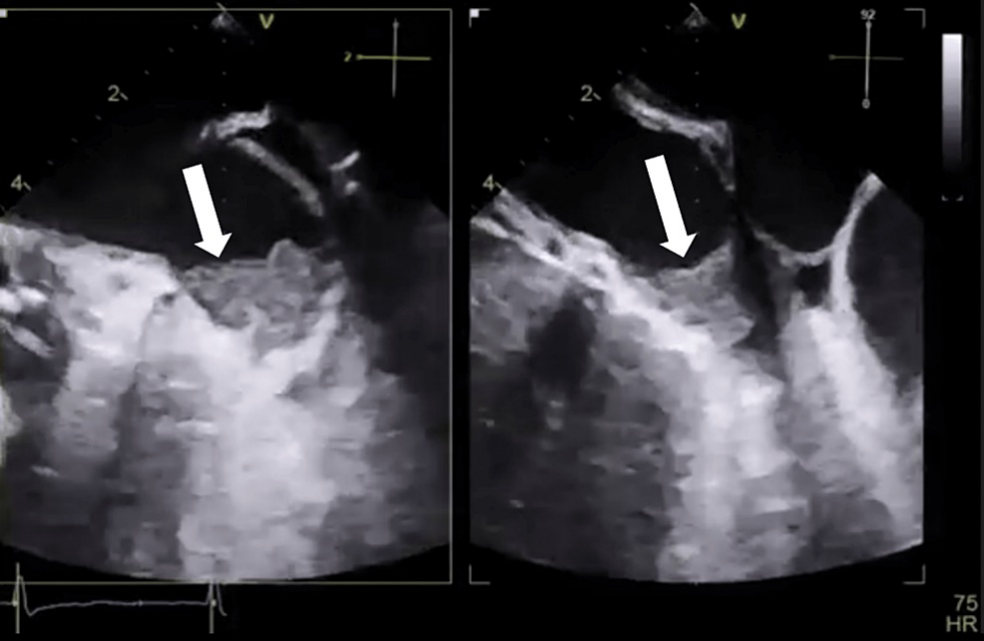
Transesophageal echocardiography mid-esophageal left atrial appendage view. Note the large thrombus occupying most of the left atrial appendage (arrow).
